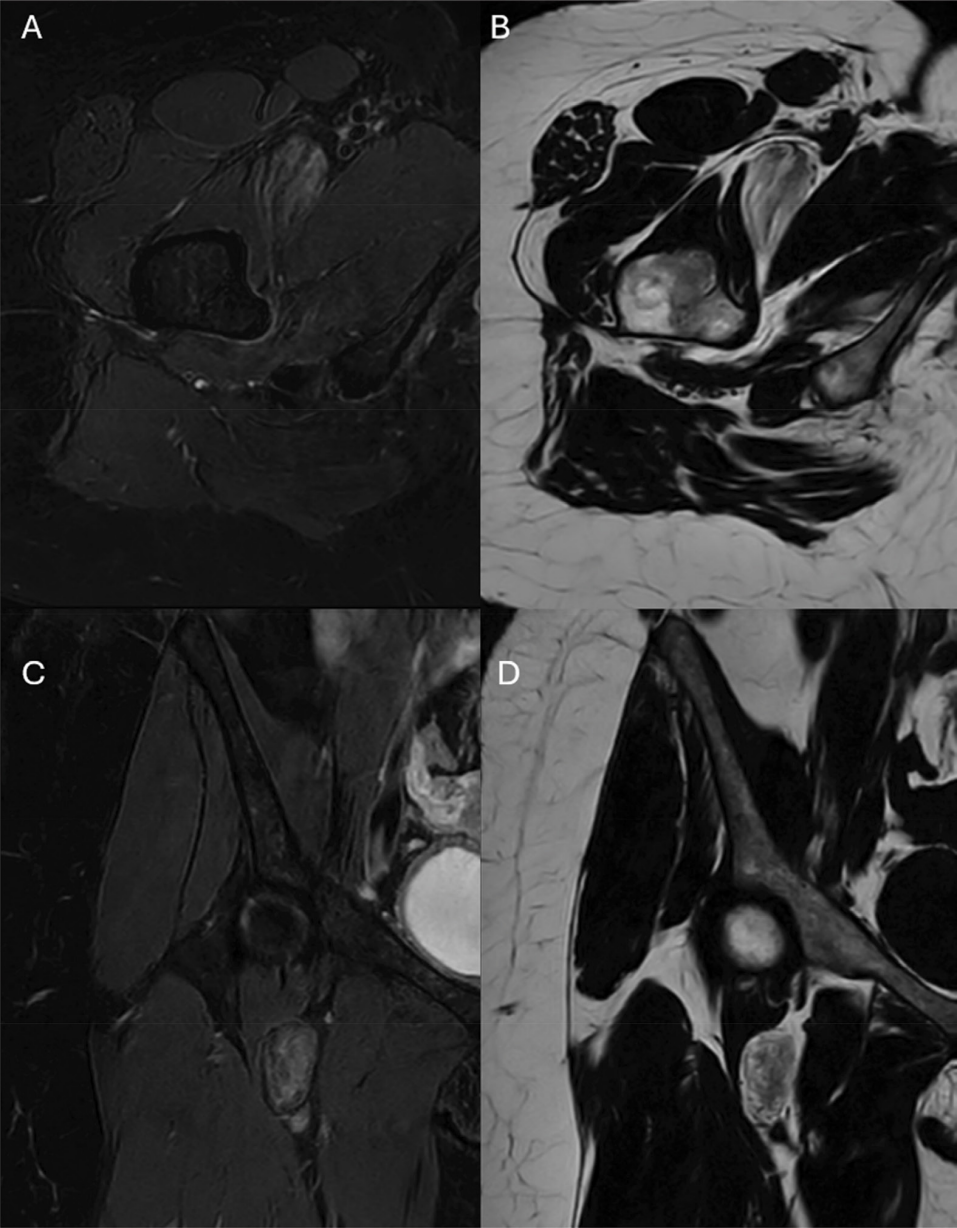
T2 Dixon MRI images demostrate the regions of high signal on the fat-only images (B and D) corresponding to low signal on the water-only images (A and C) with interspersed brush-stroke-like areas of high signal intensity on the water-only images and low signal on the fat-only images.
